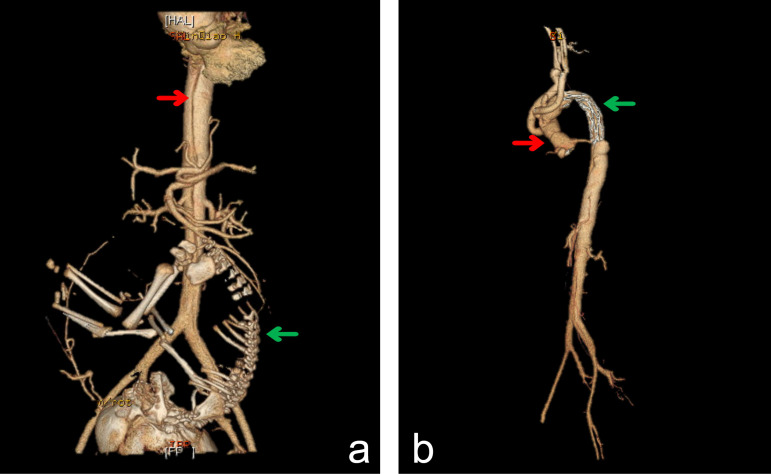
Changes in pre- and postoperative computed tomography angiography
